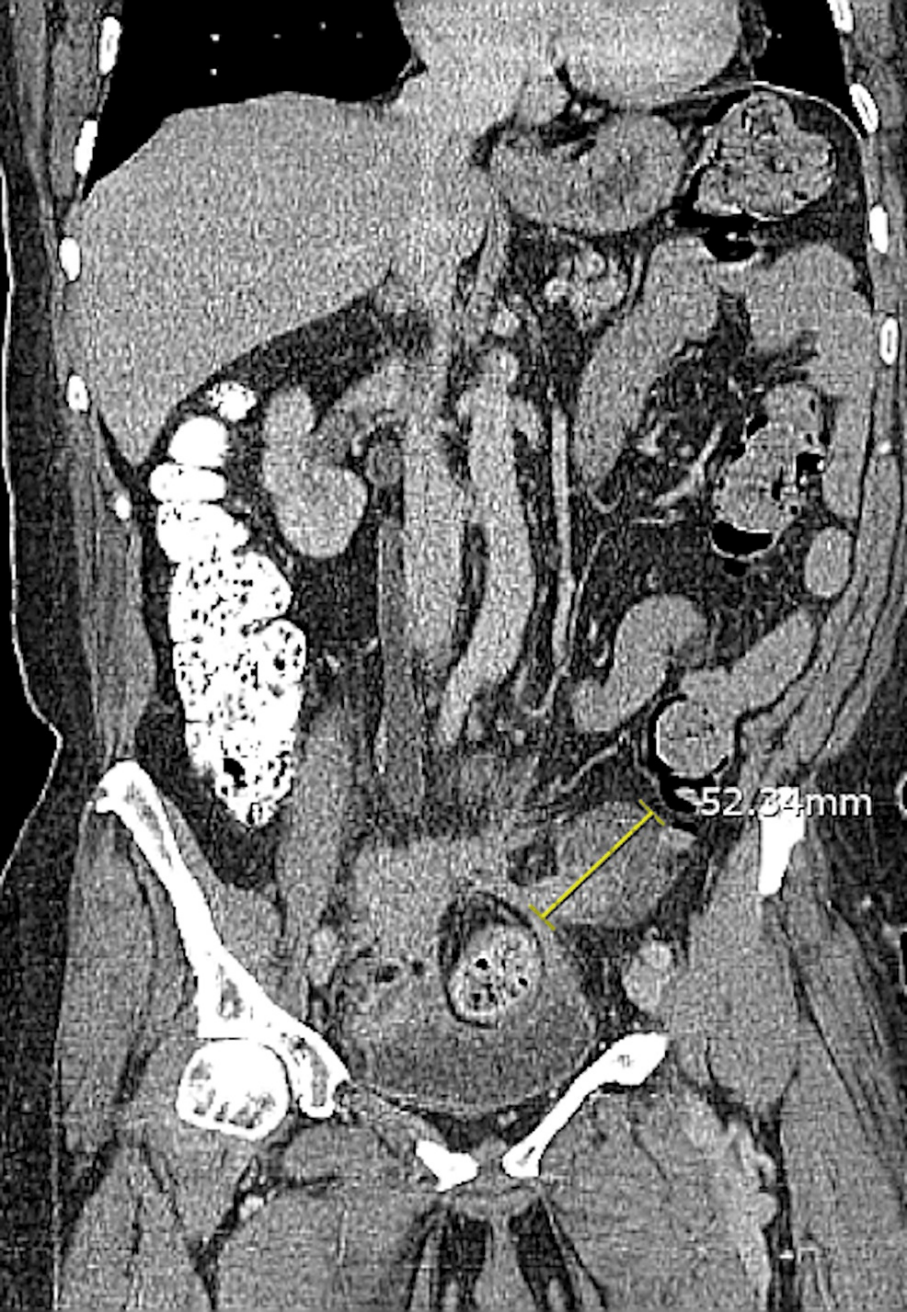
CT scan shows the stricture of the sigmoid colon by a soft tissue mass (coronal).
